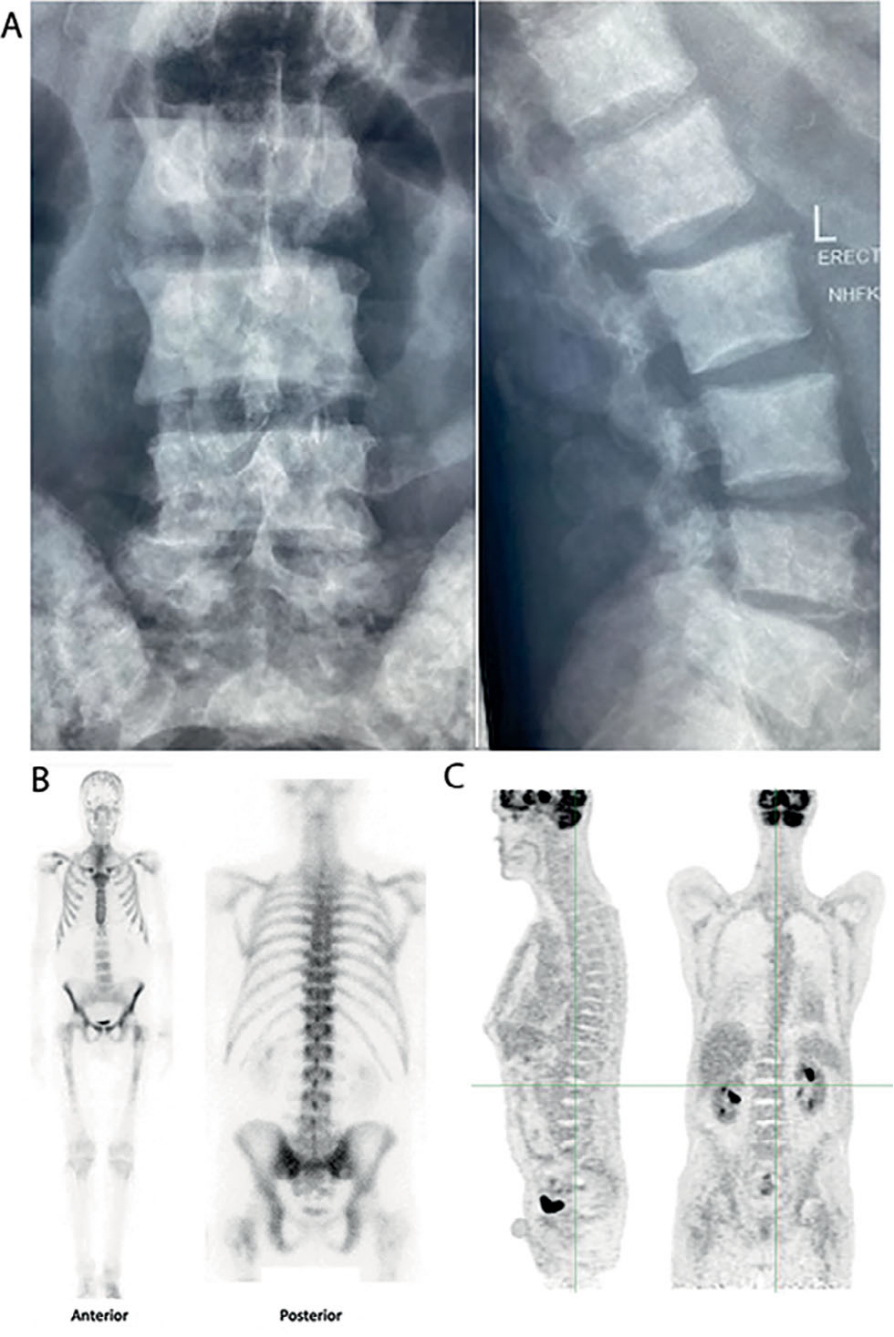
(*A*) X‐rays of lumbar spine demonstrating diffuse sclerosis with a heterogenous “mottled appearance” and no lytic lesions or fractures. (*B*) TcWBS demonstrating increased diffuse tracer uptake within skeleton compared to soft tissue. (*C*) FDG PET CT showing no focal FDG uptake to suggest a primary or secondary malignancy.
